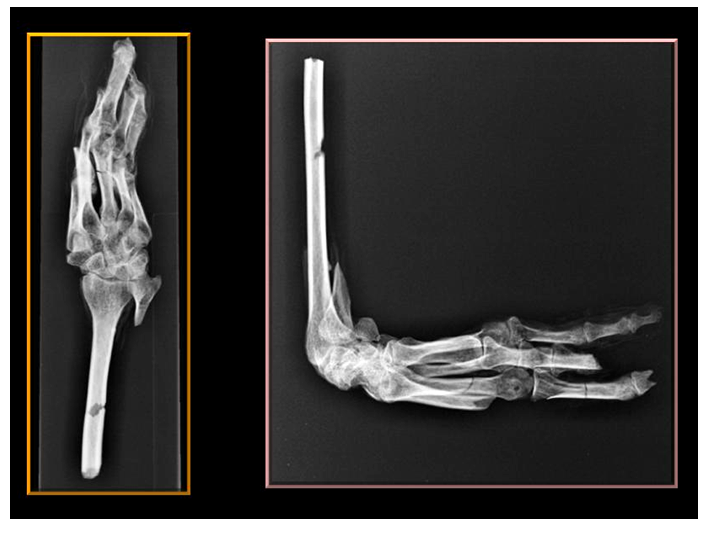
X-rays of Geheset's left hand. The images were acquired with a portable machine in the field. Several small bones show reduced density consistent with the long-standing fixed position of the deformity and disuse of the hand.
